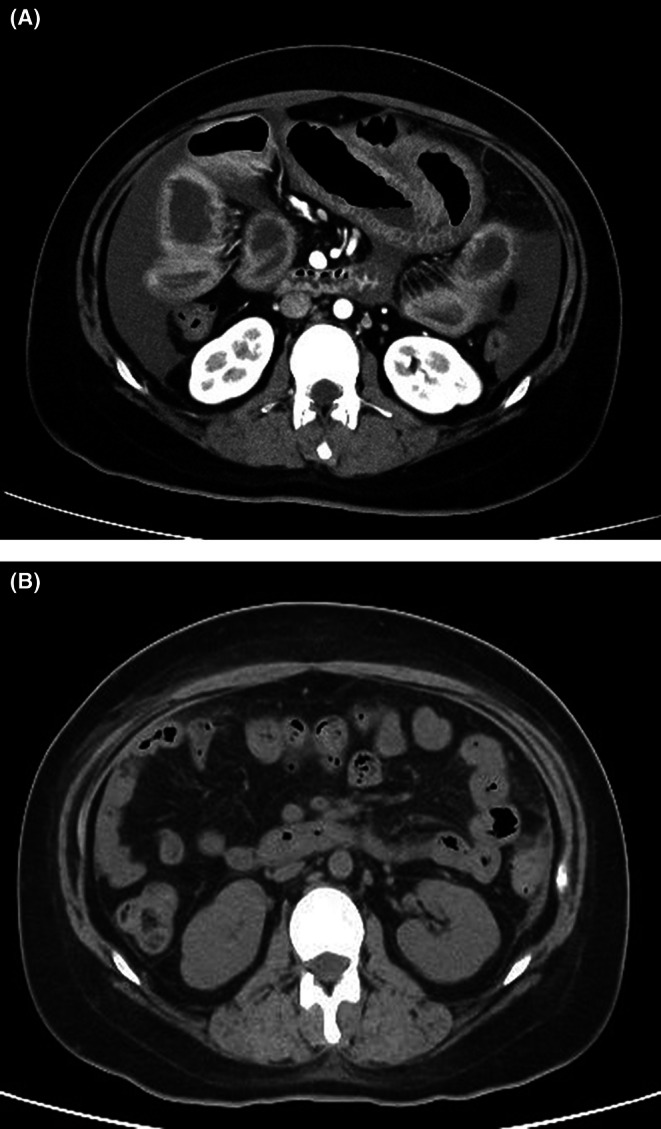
(A) Abdominal contrast‐enhanced computed tomography showed diffuse edema of the small bowel wall with ascites, raising the suspicion of angioedema or lupus enteritis. (B) Follow‐up CT images demonstrated the resolution of intestinal edema after treatment.
